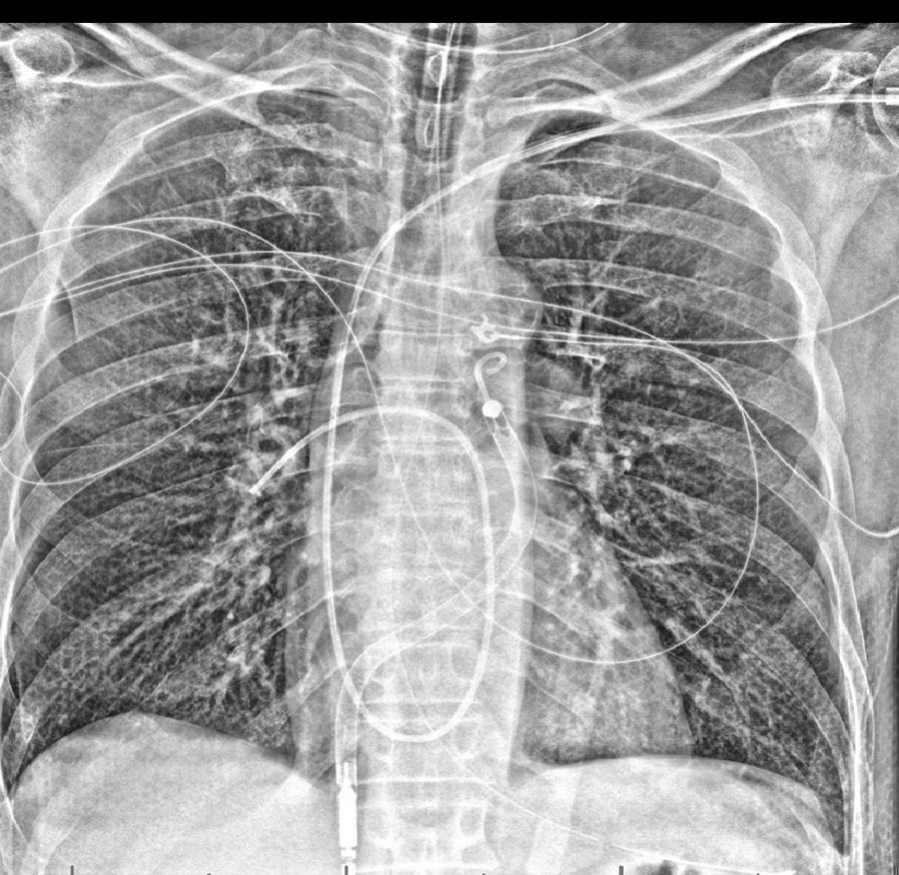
Chest radiograph with CLAHE filter in a patient with an Impella RP for right ventricle support. The pigtail portion in the outflow is in the main pulmonary artery. The inlet is in the IVC (white arrow). (CLAHE—contrast limited adaptive histogram equalization, IVC–inferior vena cava)
